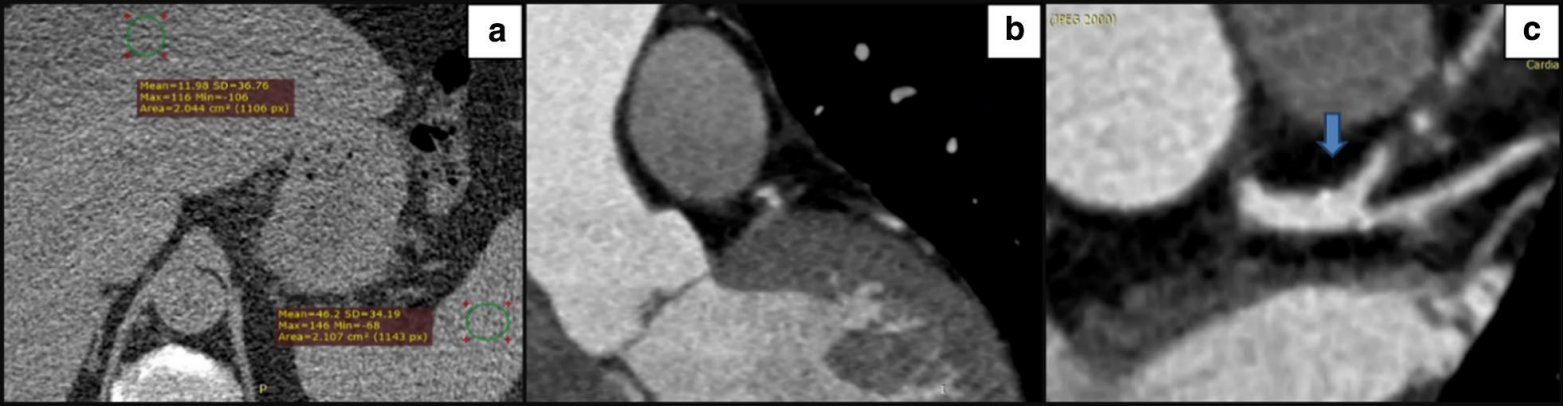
A male patient 67 years old, hypertensive, diabetic, and smoker. a Axial non-enhanced CT demonstrates attenuation measurements of the liver and spleen with a mean liver attenuation of 11.9 HU and a mean spleen attenuation of 46.2 HU. b Coronary CT angiography images shows a non-calcified plaque demonstrating a napkin-ring sign with a central low-attenuation area, surrounded by a peripheral rim of higher attenuation. c Coronary CT angiography images shows a calcified ostial lesion with spotty calcifications (arrow) of left main coronary artery
